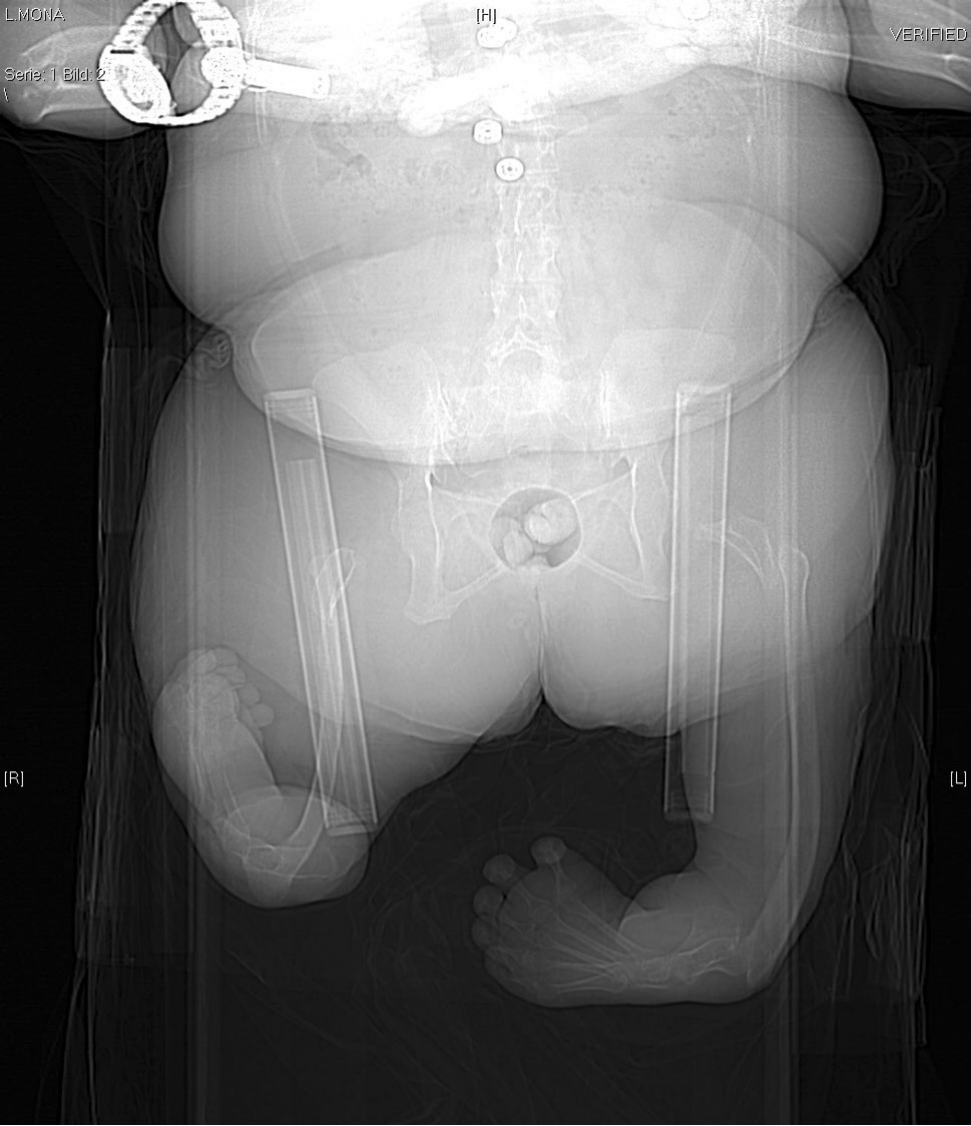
Computed tomography scan of one study participant with bilateral proximal focal femoral deficiency and fibular hypoplasia and tibial aplasia of the lower limbs
